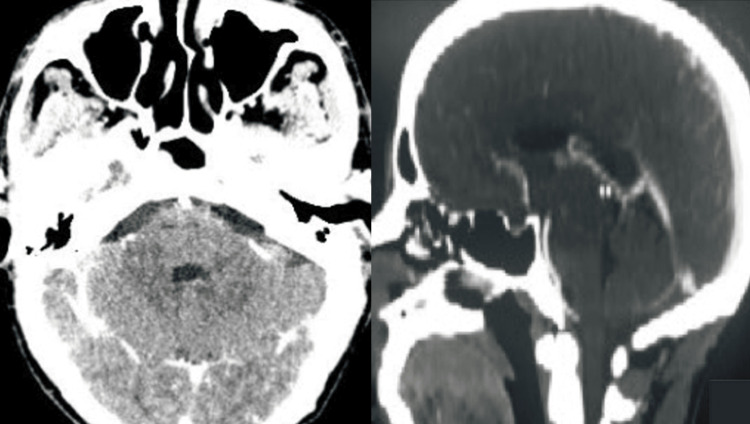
Follow-up neuroimaging findings Post-treatment axial cerebral CT images show a marked reduction in the size of the pontine lesion. The previously observed ring enhancement has resolved, consistent with a favorable therapeutic response. CT: computed tomography.
